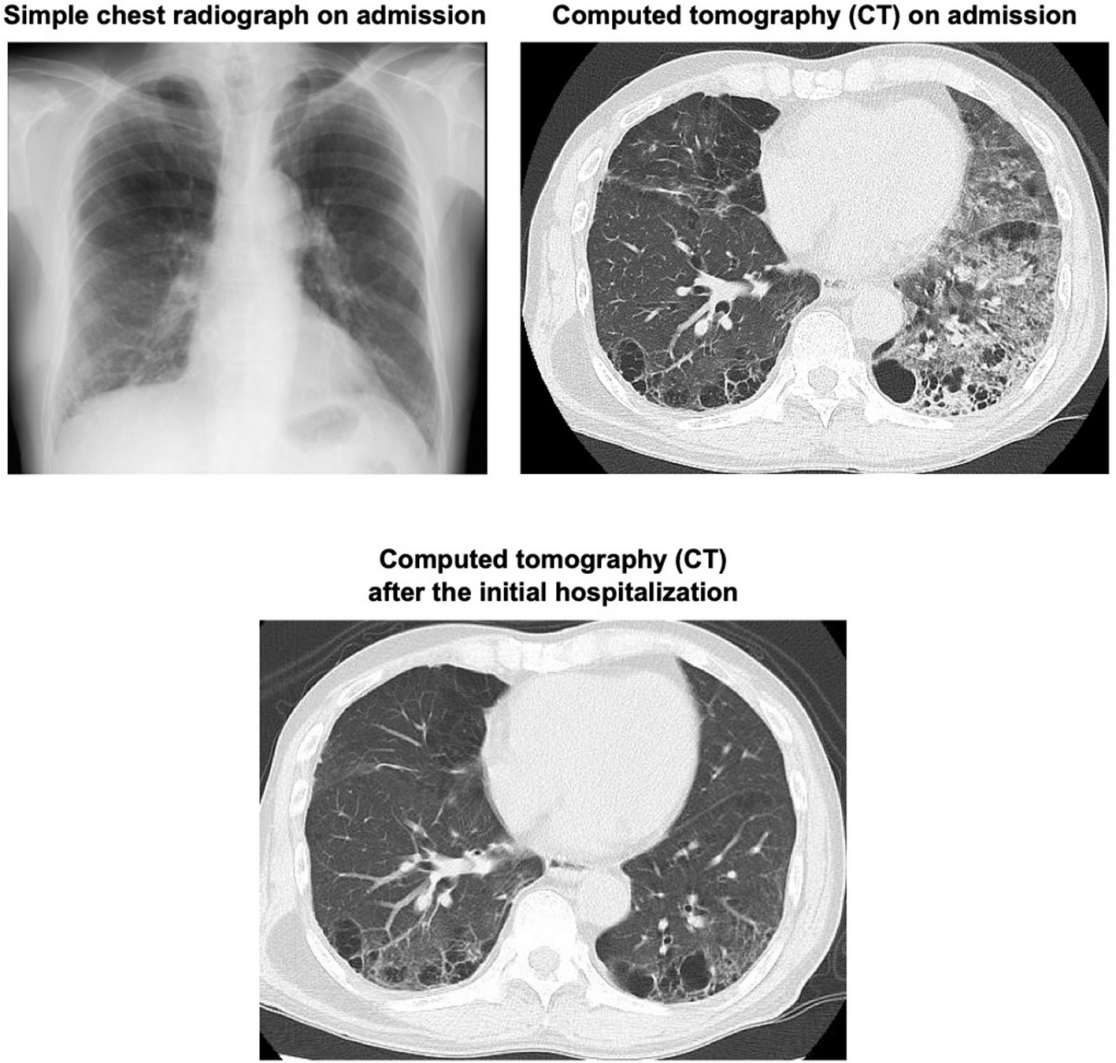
A simple chest photograph at the time of emergency transport showed reticular shadows in the bilateral lower lung fields (upper left panel). Computed tomography (CT) at the time of emergency transport showed an infiltrative shadow with air bronchograms in the right lower lobe. There were emphysematous changes in the bilateral lungs (lower right panel). CT performed 106 days after initial discharge showed that the infiltrative shadow in the right lower lobe had disappeared (lower panel).
